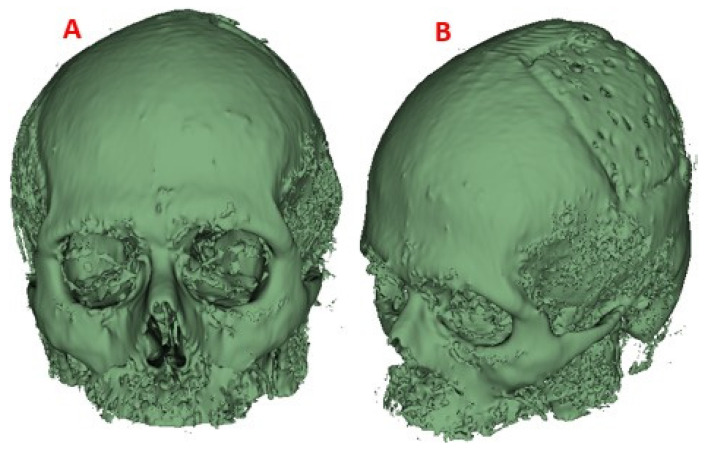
CT scan of the patient, with the custom PMMA implant, 14 days post-surgery: (A) Image of the skull with the PMMA implant attached, front view; (B) Image of the skull with the attached PMMA implant, 3D view.
